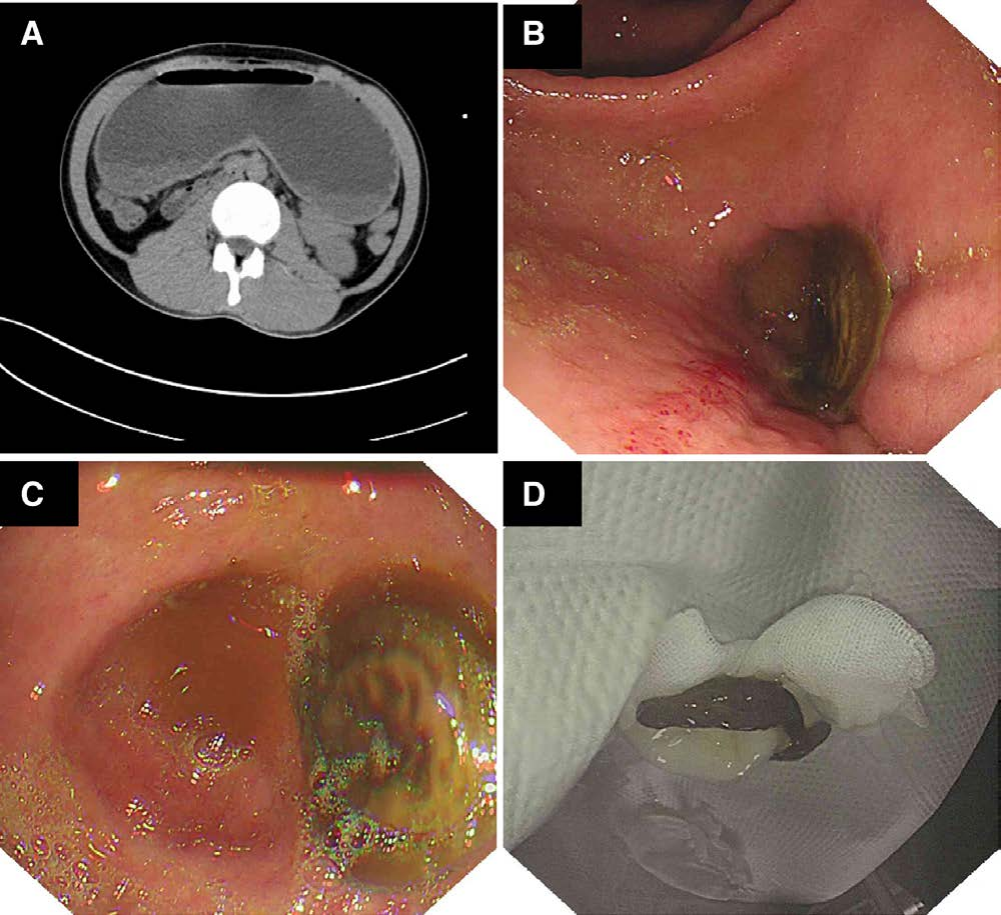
(A) The emergency CT images of the patient. (B and C) The gastroscope images of the posterior wall of the patient’s posterior antrum. (D) The in vitro observation of the pyloric obstruction.
